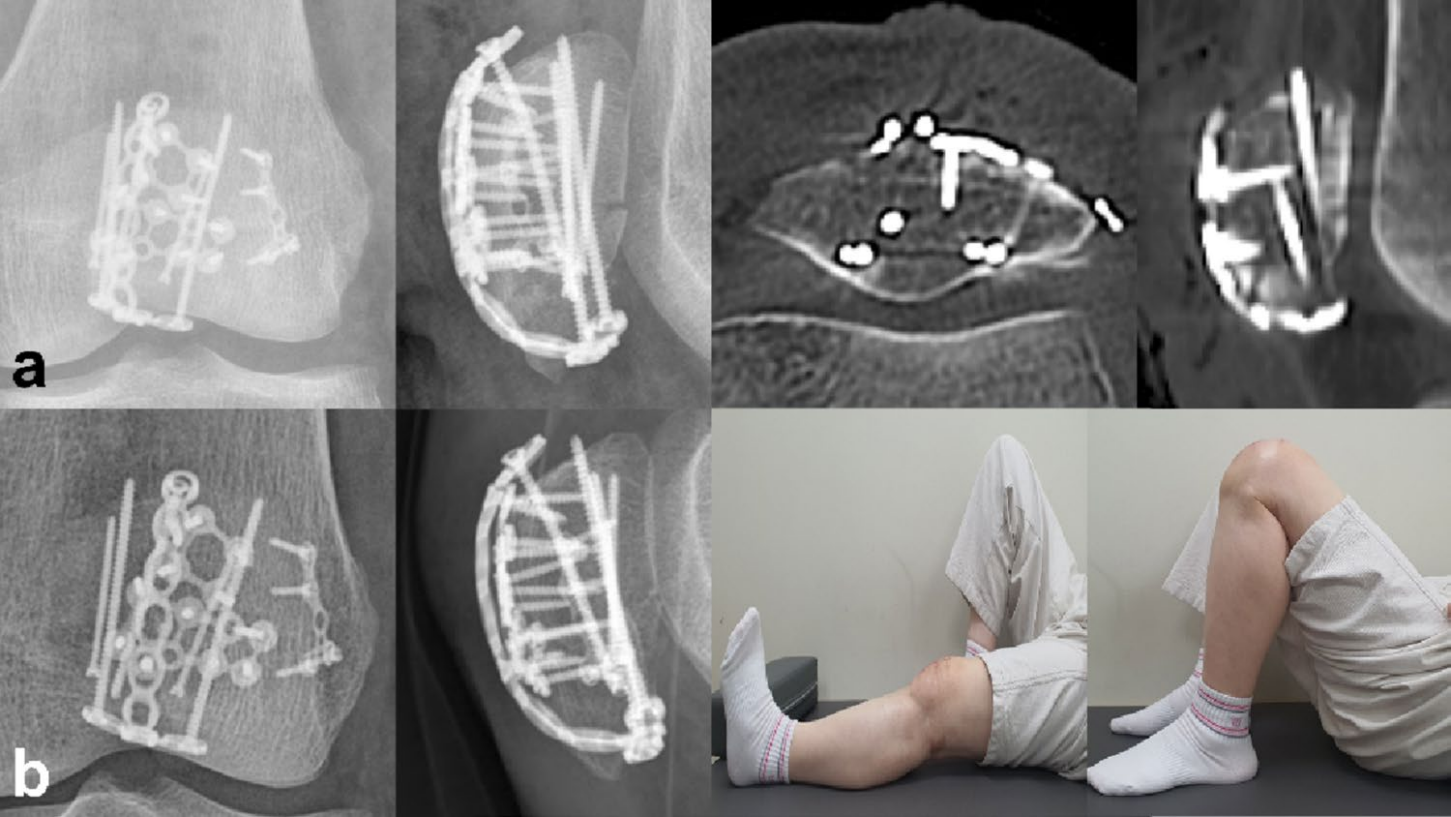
a Postoperative radiographs show the tension-band plating with multiple mini plates. All identified fragments were anatomically stabilized. There was no articular step-off showing anatomical reduction. b After 6 months, the radiologic bony union was achieved, and the range of motion was fully recovered
